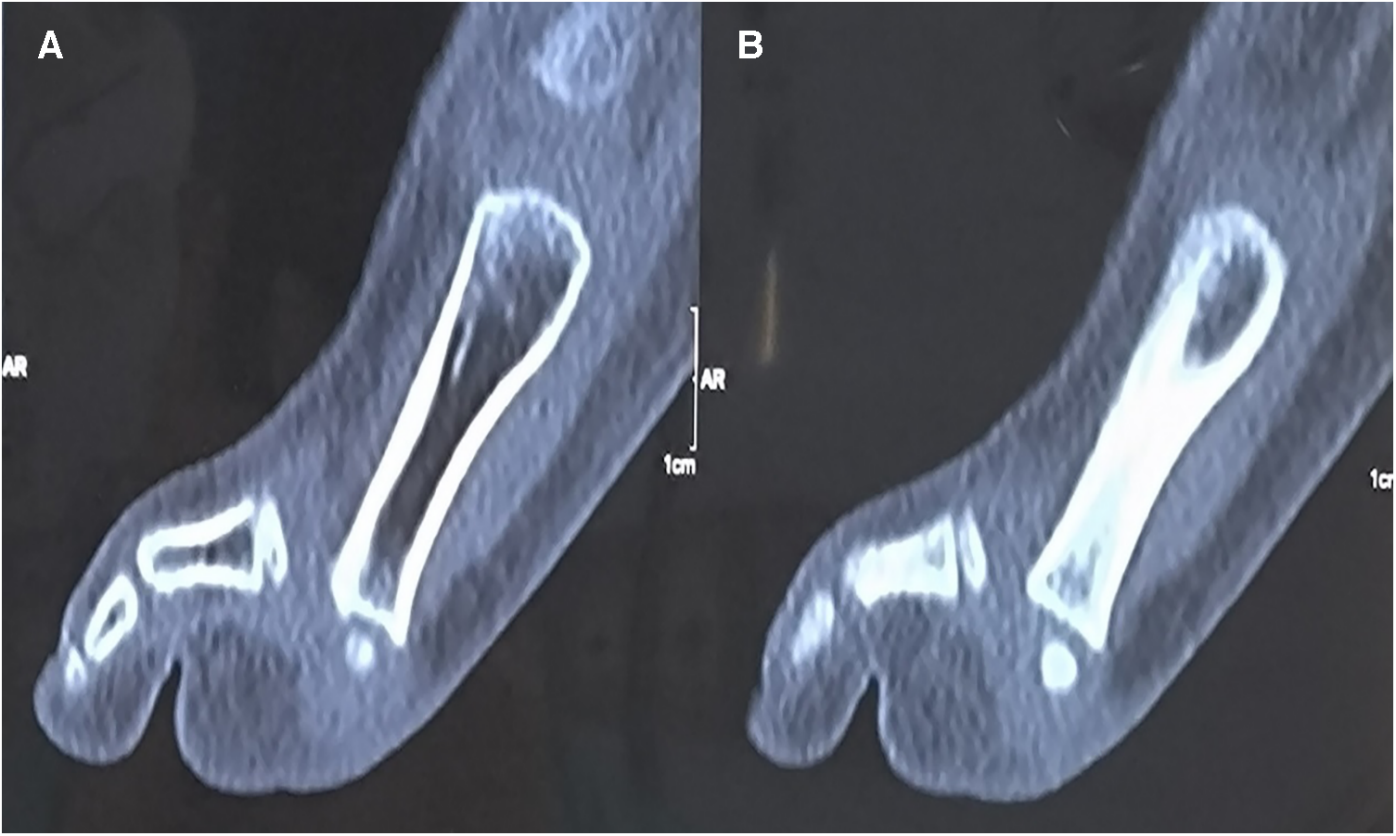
(A, B) Computed tomography shows the dislocation of the fifth MTPJ in the sagittal image.
